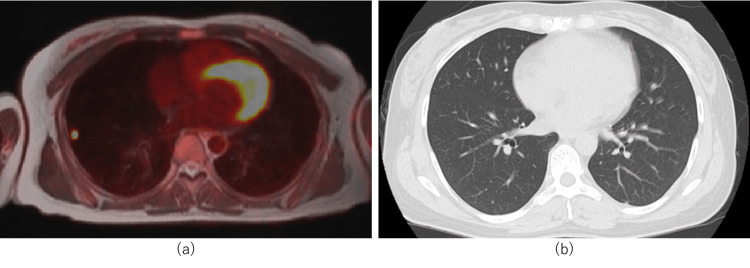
PET-CT and contrast CT of the lung (a) A positron emission tomography (PET)-CT scan revealed a nodule with a high uptake in the right lung; (b) a contrast CT scan revealed no obvious nodule in the right lung
